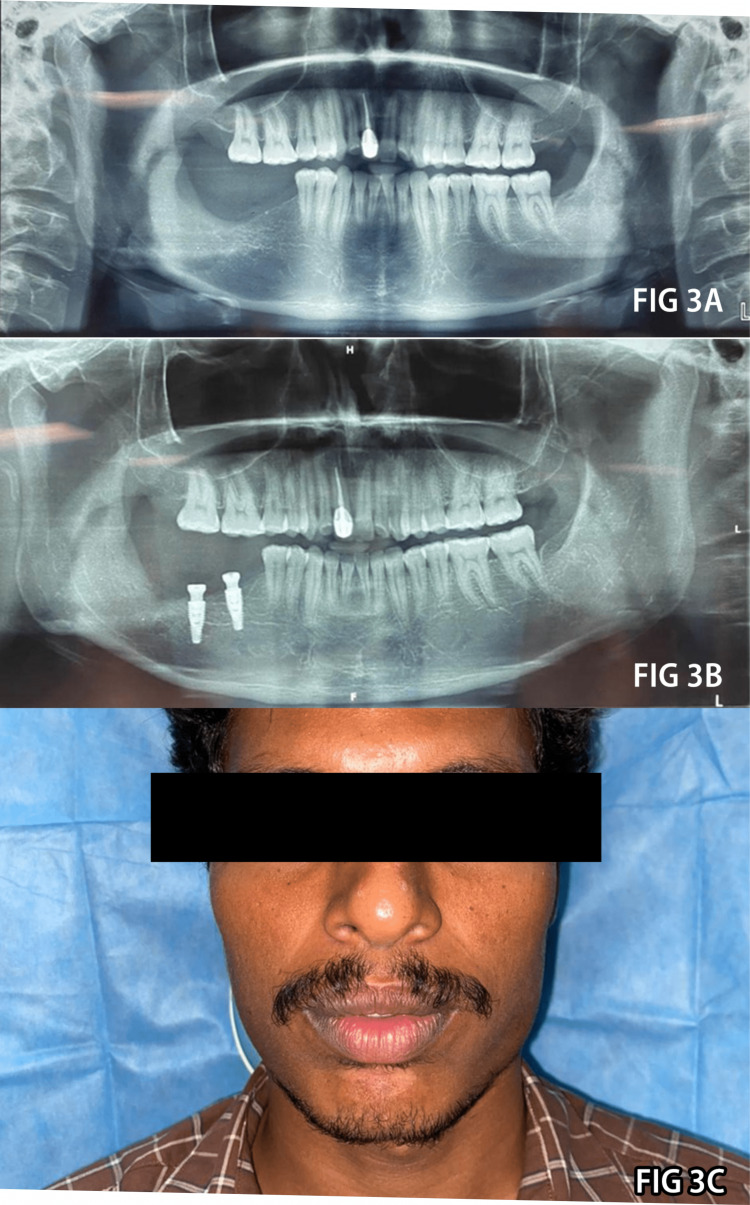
Rehabilitation phase. A: Panoramic radiographic image represents full bone regeneration after 24 months. B and C: Radiographic and clinical profile after implant placement.
